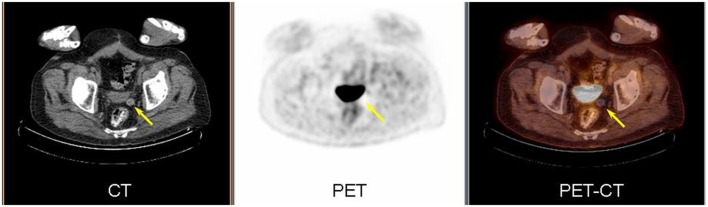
PET/CT images demonstrate the soft tissue lesion has moved from the right to left hemipelvis (arrow) on low dose CT (left). The lesion demonstrated no metabolic activity on PET (center) and fused image (right).
